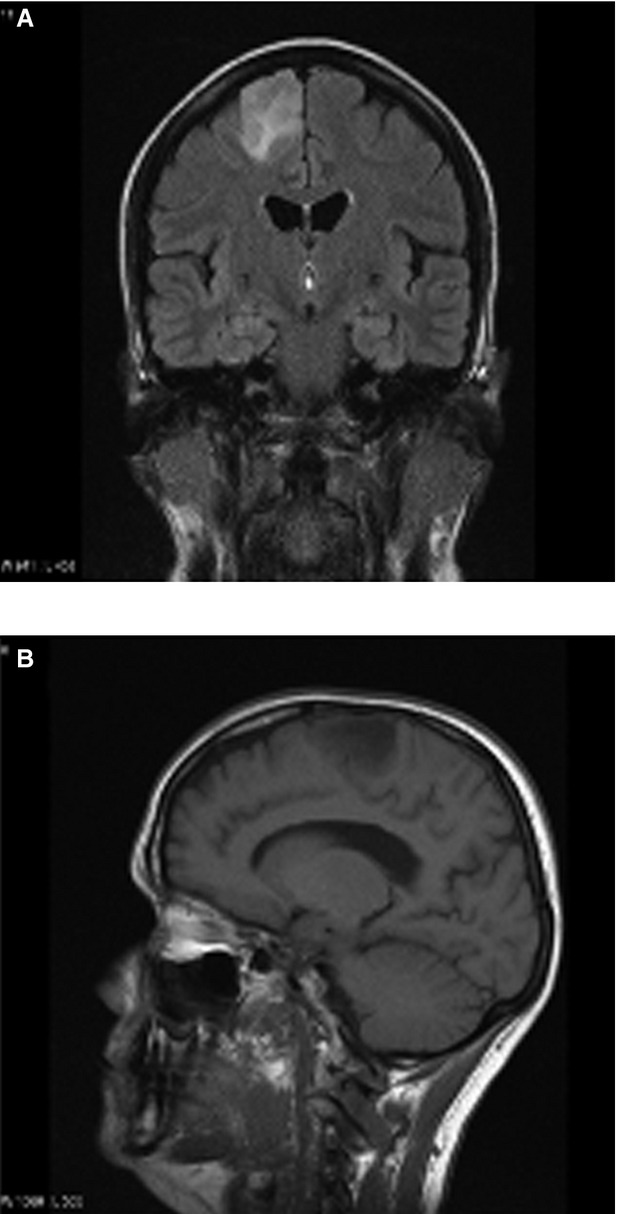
Coronal FLAIR (A) and sagittal T_1_-weighted (B) MR images of a low grade glioma in right superior frontal gyrus, causing refractory focal epilepsy.
